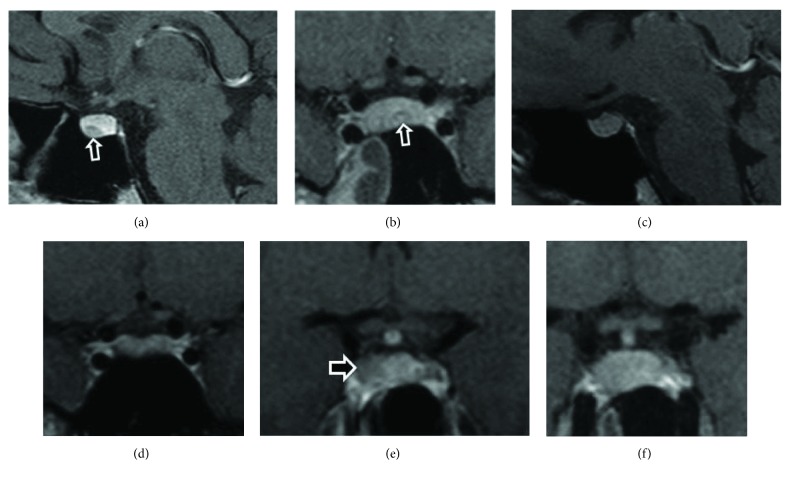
Pituitary MRI scans of the proband's sister (case 3), showing a Rathke's cleft cyst when first screened at the age of 17 (a, b), which completely disappeared in the following 5 years (c, d). A 4 mm microadenoma became visible in the right side (e) of her bulky pituitary (f).
